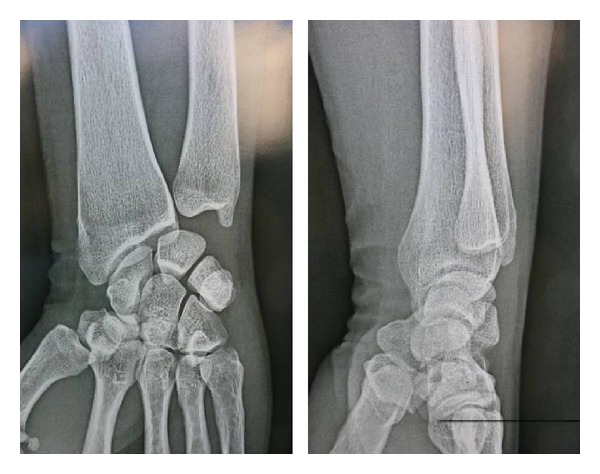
X-ray of the hand shows no bony abnormality.
